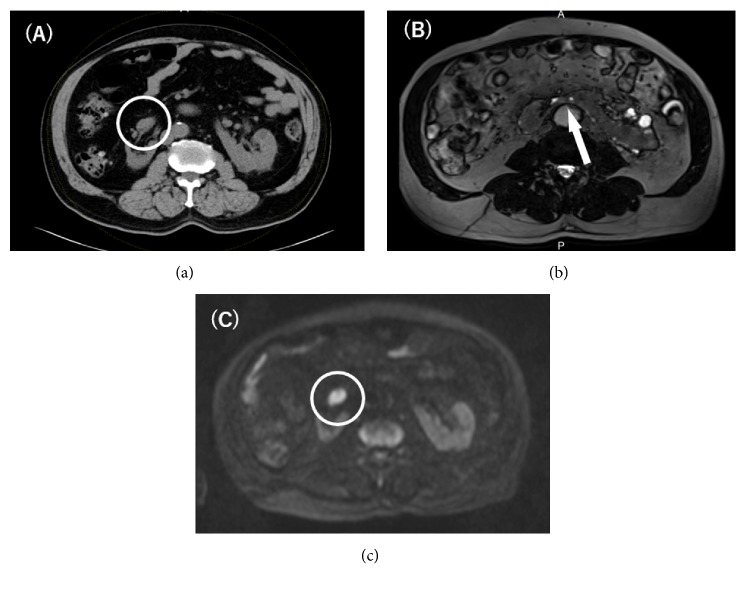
(a) CT scan axial image. A 28 mm tumor is shown in the right renal pelvis. (b) T2-weighted MR image.* Arrow* indicates the isthmus of a horseshoe kidney. (c) Diffusion-weighted MR image. The tumor in the right renal pelvis shows high signal.
